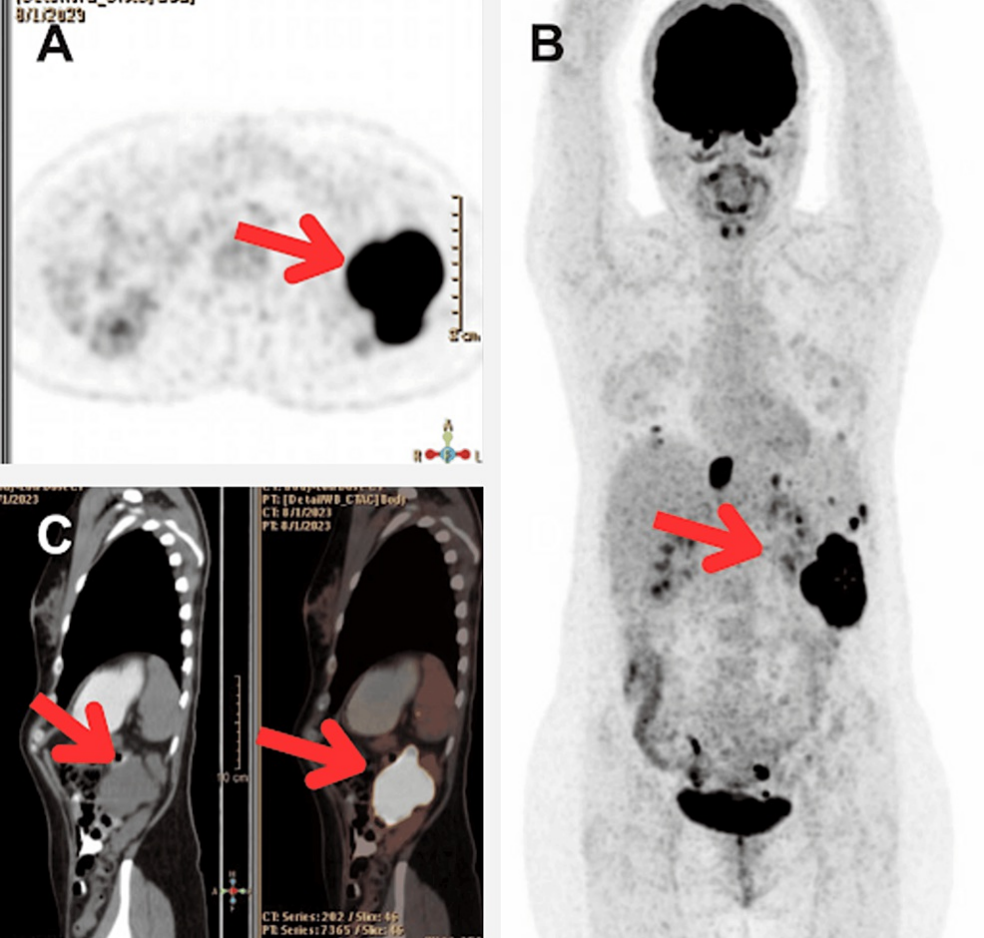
Abdominal positron emission tomography-computed tomography (PET-CT) In the three panels (A: axial in grayscale, B: coronal in grayscale, and C: simple phase contrast in hot metal scale), a left flank topography reveals the presence of a space-occupying lesion with lobulated contours, partially defined, in close contact with loops of the small intestine (proximal ileum and distal jejunum) and the spleen. The lesion measures 106 x 63 x 100 mm, with a volume of 350 cm³, and exhibits heterogeneous density, showing areas with hematic density (average attenuation of 75 HU), with suggestive images of peritoneal implants.
